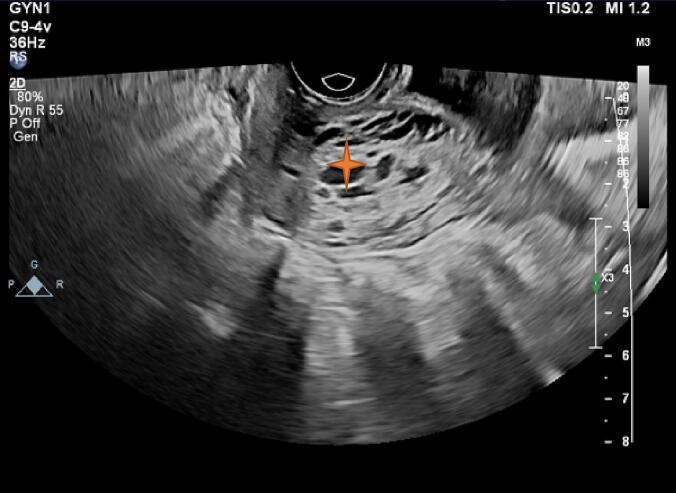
Transvaginal sonography, hypoechoic areas were suggestive of varicose veins, and fully active vessels were seen in the cervical region (star), especially in the anterior lip, which continued to the cervix.
